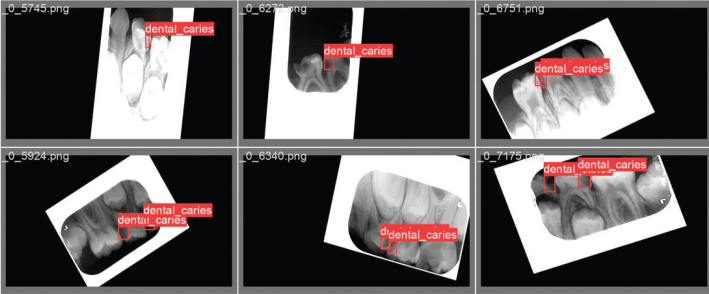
Radiographic image samples labeled by a pediatric dentist.
